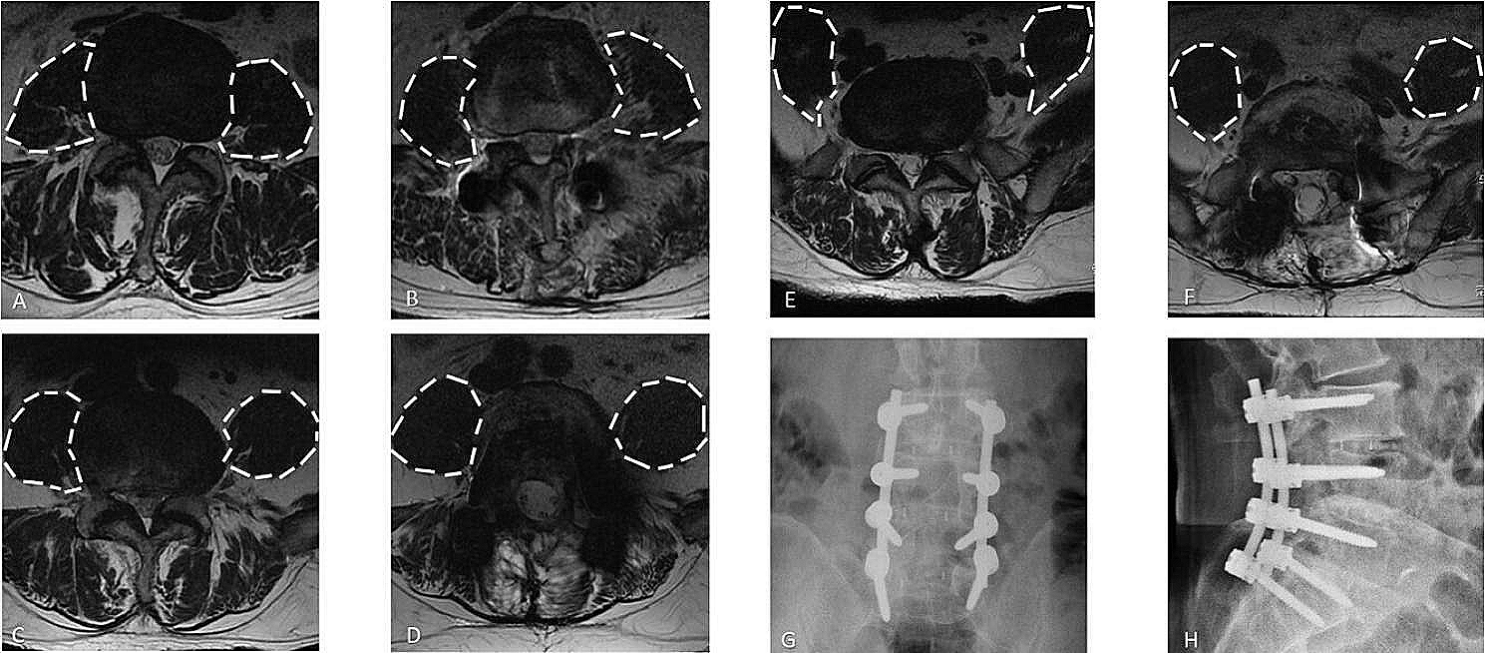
Preoperative and postoperative radiographs of a 69-year-old man presenting with first sacral vertebra (S1) screw non-loosening at the 38-month follow-up (G, H). Preoperative functional cross-sectional areas (fCSAs) of the psoas major muscle (PS) at L3–4 (A), L4–5 (C), and L5–S1 (E) are shown. Postoperative fCSAs of the PS at L3–4 (B), L4–5 (D), and L5–S1 (F) are shown. The ratio of postoperative and preoperative rfCSA at L3-S1 was 1.50, which was higher than the proposed 1.075 cutoff value
